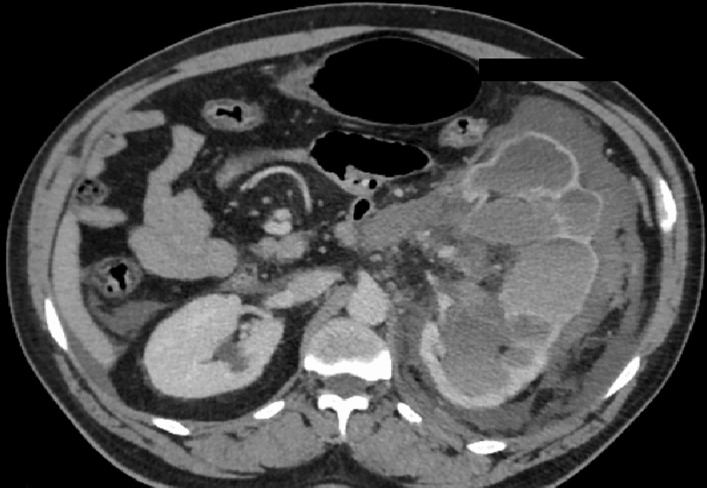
Abdominal computed tomography scan showed a high-volume retroperitoneal hematoma and multiply lacerated lower pole of the left kidney
